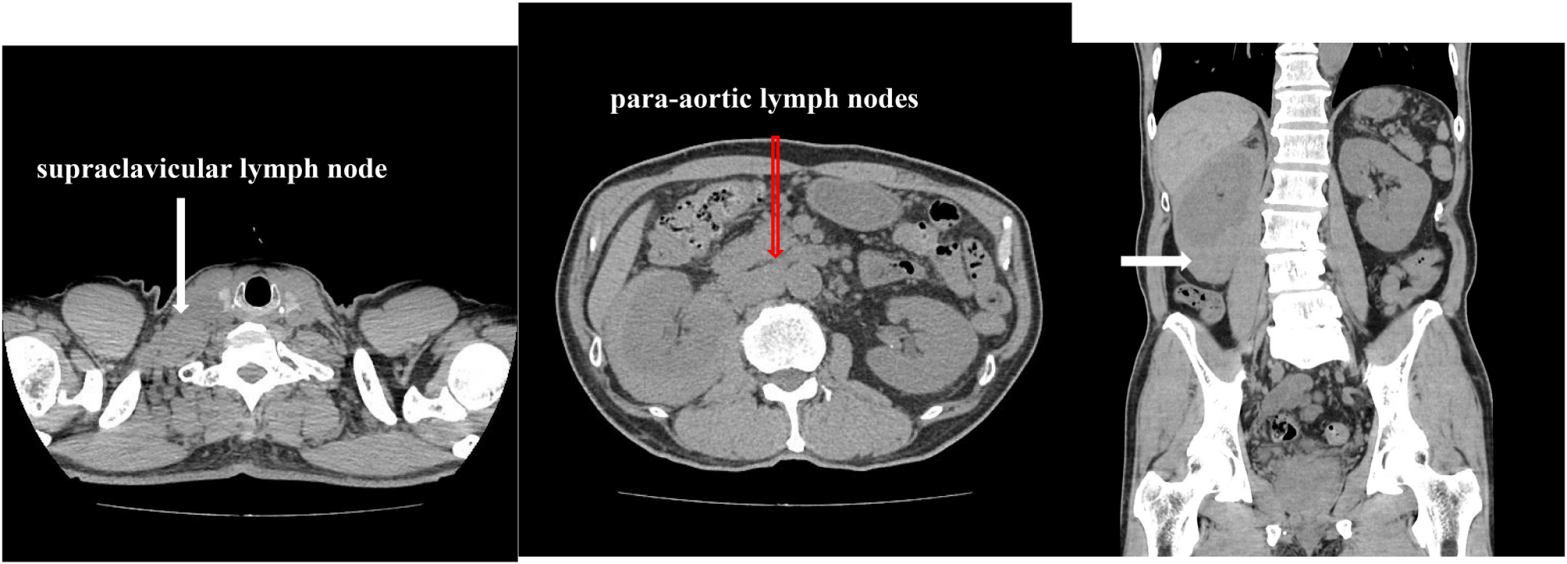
Chest plain computed tomography(CT) did not reveal any abnormal findings in the lung field but did reveal an enlarged right supraclavicular lymph node (⇩). Plain abdominal CT revealed a tumorous lesion around the right kidney. The tumor had a clear border and was homogeneous inside, with the same density as the renal parenchyma (⇨). In addition, enlarged para‐aortic lymph nodes were also observed (⇩).
